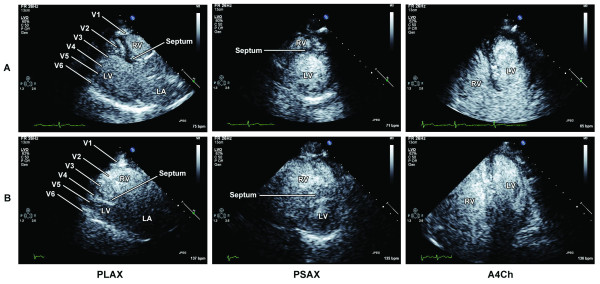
Parasternal long axis (PLAX), parasternal short axis (PSAX), and apical 4 chamber (A4Ch) views at rest (A) and stress (B). In the PLAX view during the stress phase, note the marked right ventricular enlargement into the region typically covered by leads V1-V3.
